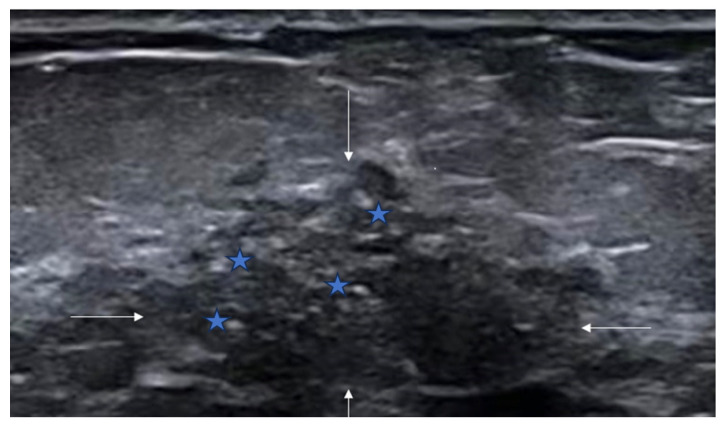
Ultrasound features of non-mass breast lesions of Type I. A case of invasive ductal carcinoma. Ultrasound reveals mottled hypoechoic areas within the breast (indicated by the white arrows), scattered hyperechoic foci (indicated by blue five-pointed star).
